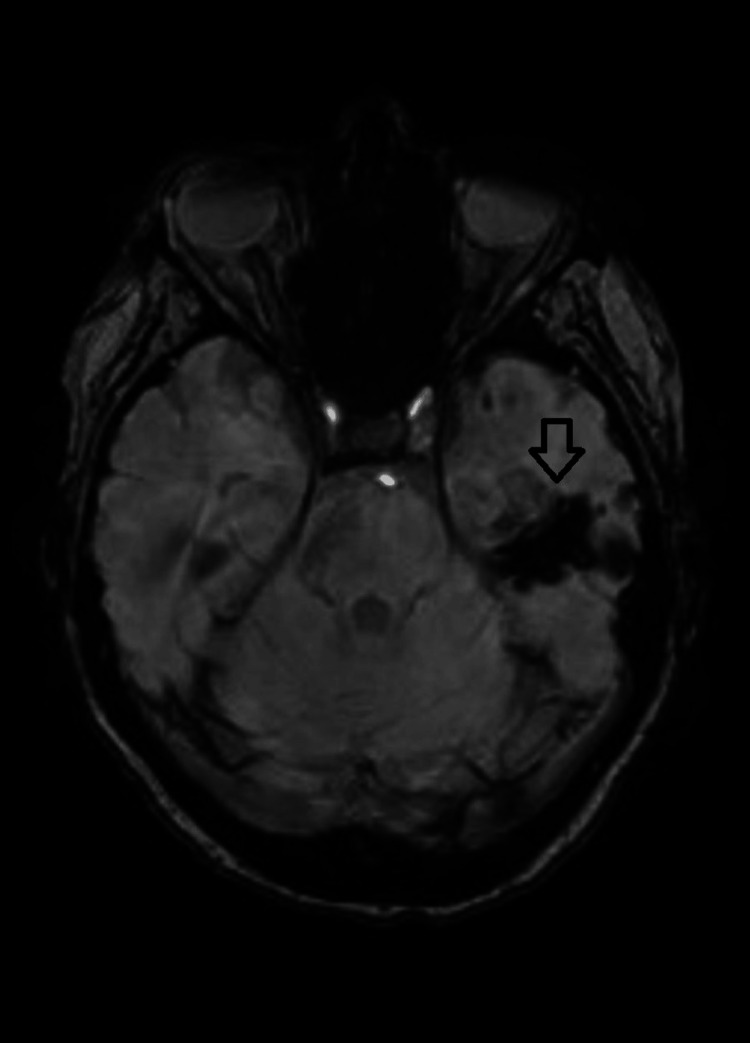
Magnetic resonance image with contrast done four weeks post-surgery showing the healing progress of the abscess and no recollection
